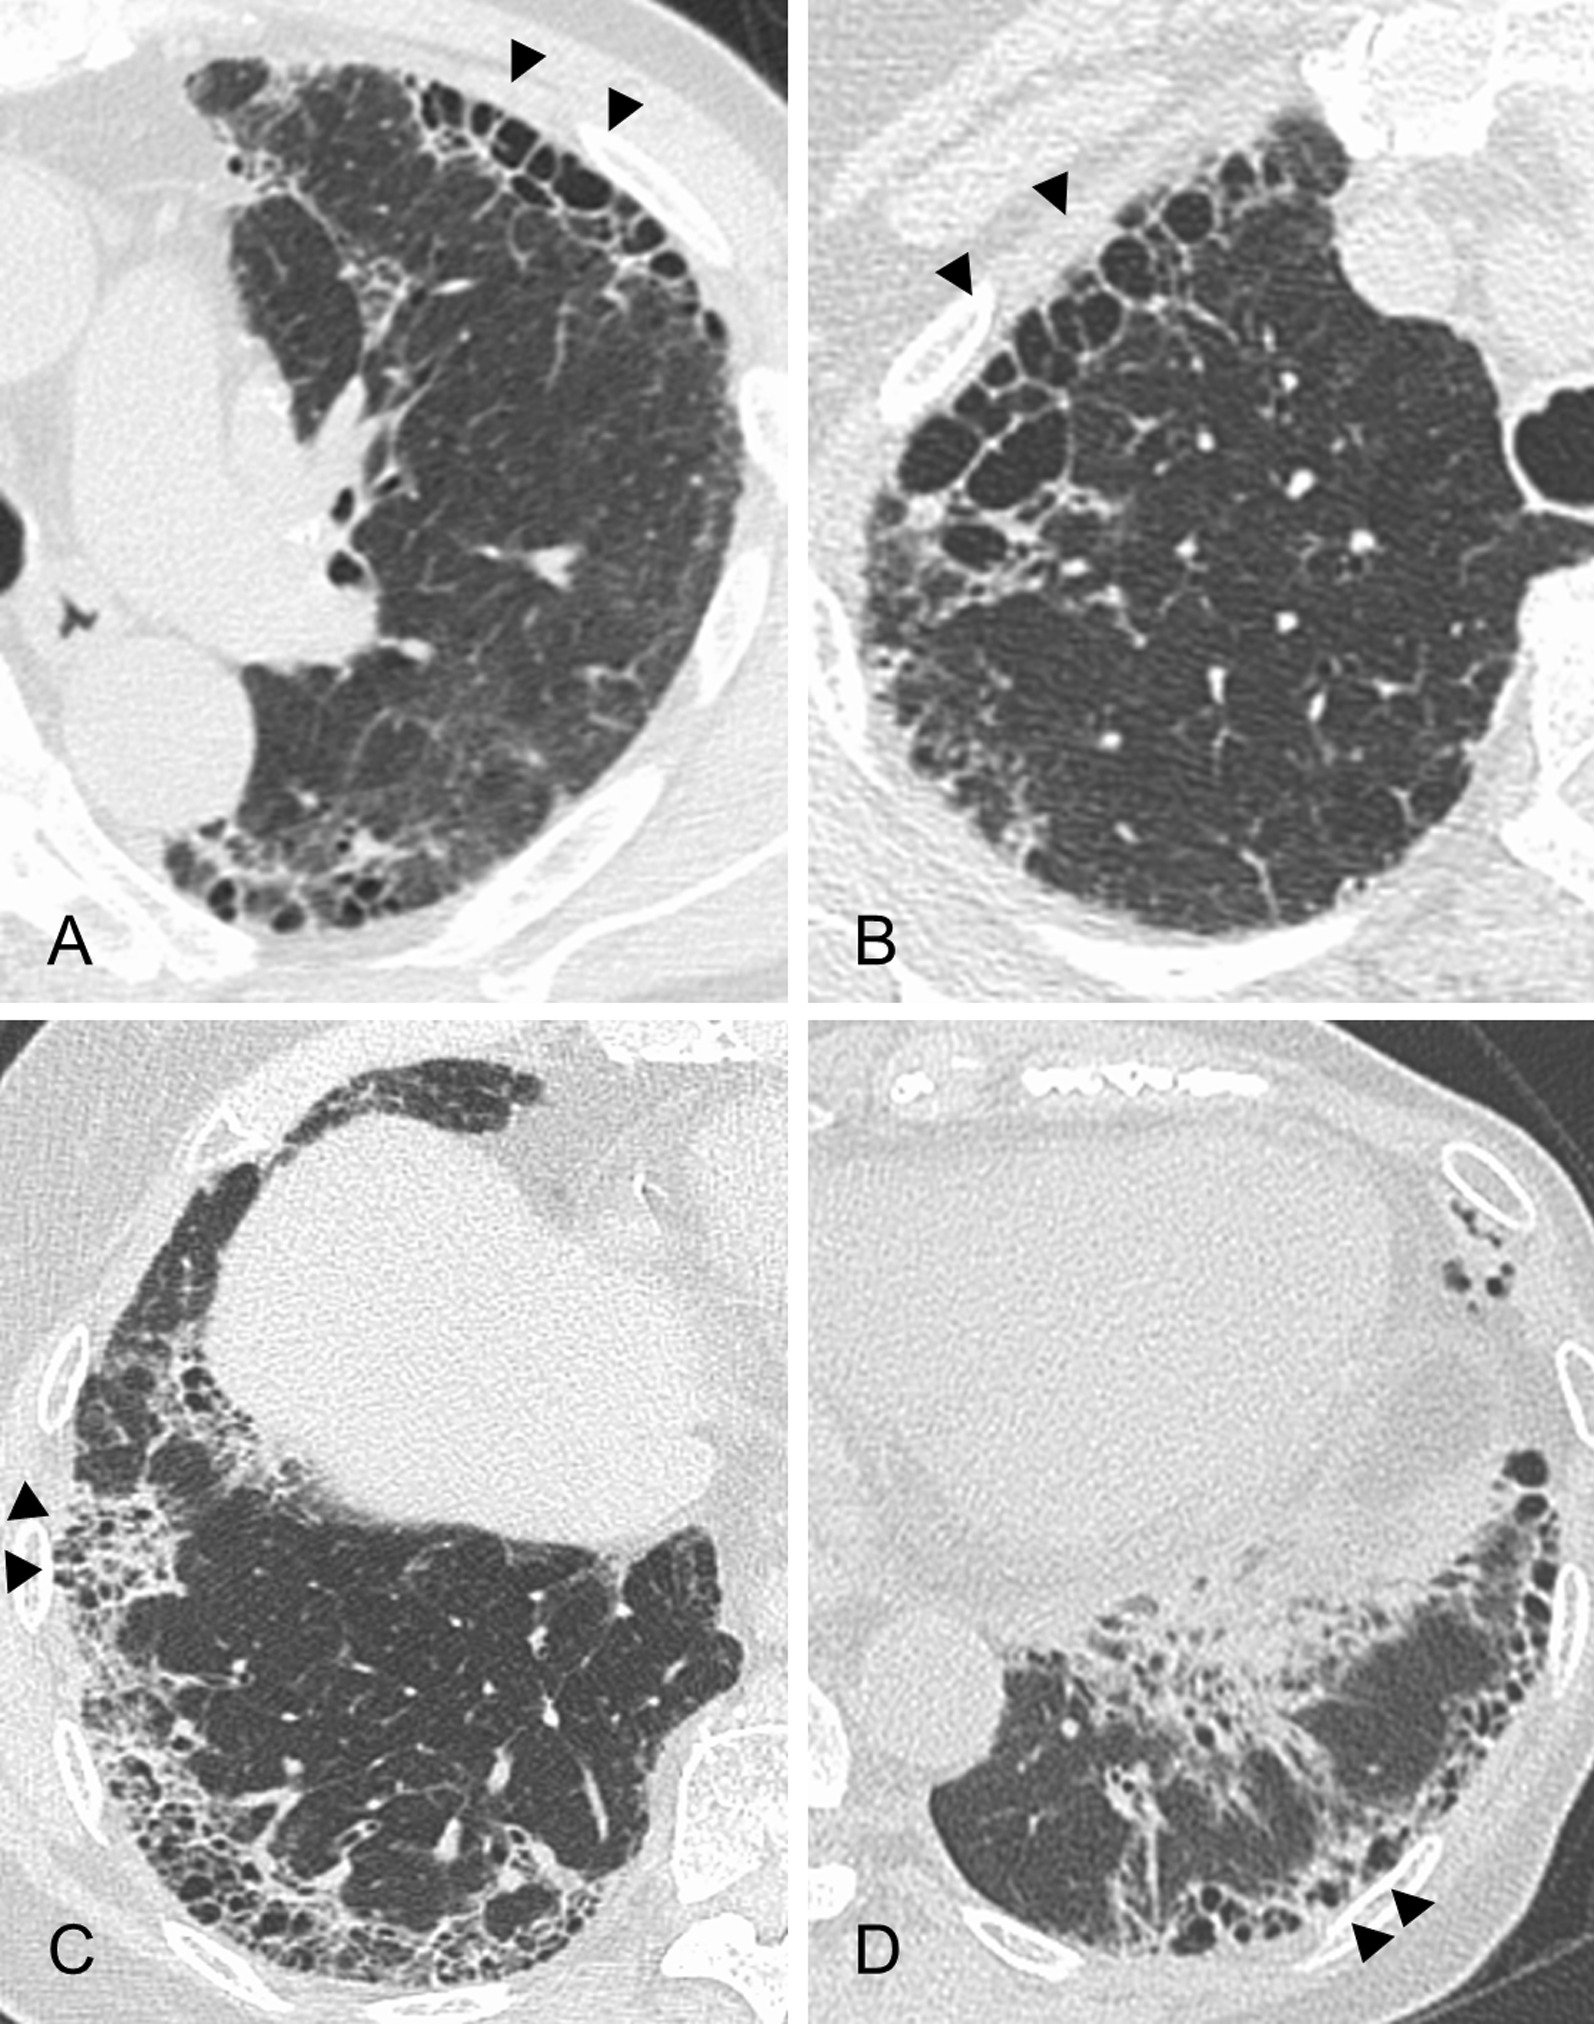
A, B High-resolution computed tomography scans of anterior upper lobe honeycomb-like lesions showing a concentration of cystic air spaces within the anterior aspect of the upper lobes (arrowheads). A A 69-year-old man. B A 72-year-old woman. C, D High-resolution computed tomography scans of increased attenuation around honeycomb or traction bronchiectasis (arrowheads). C A 77-year-old woman. D A 73-year-old woman
